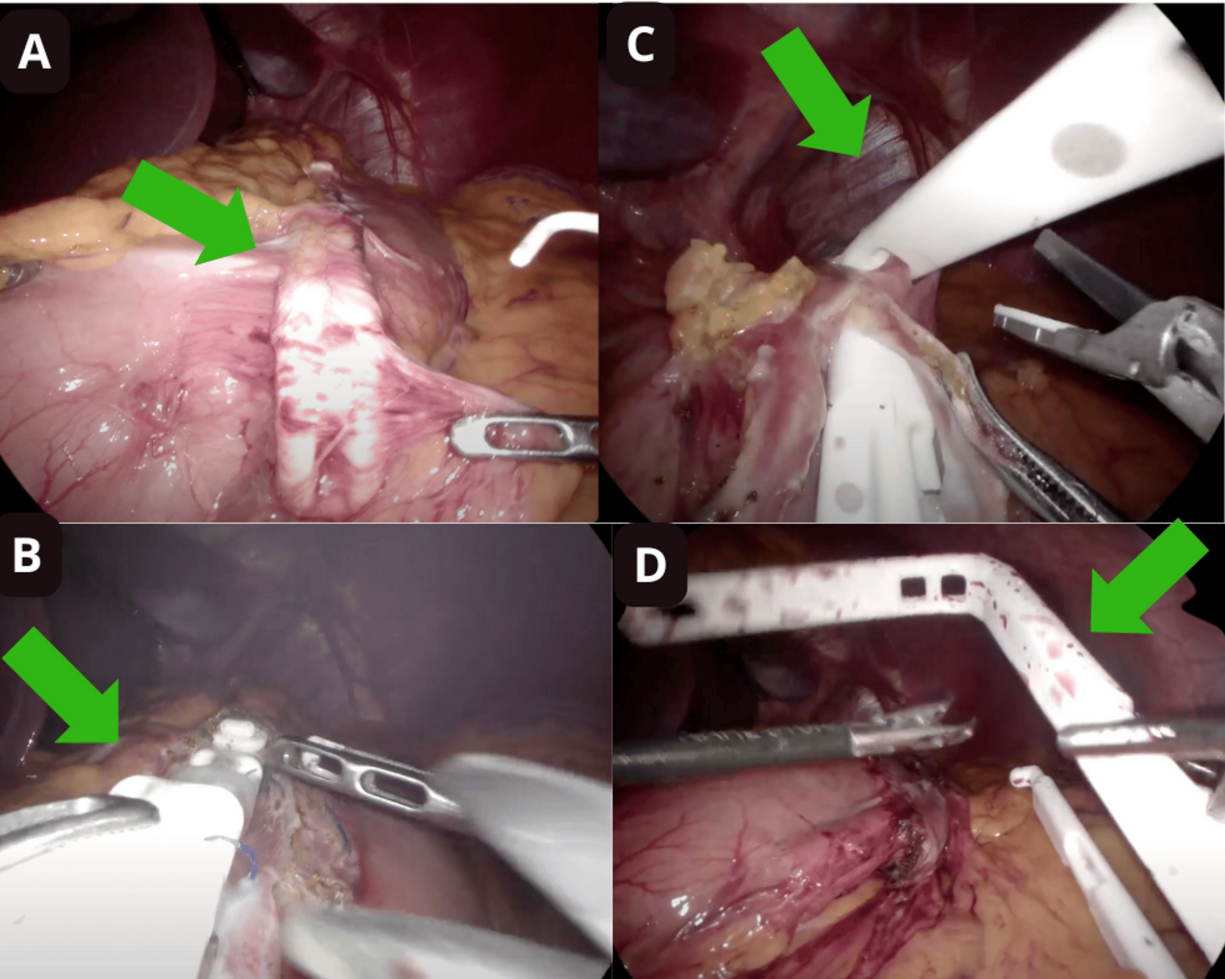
Laparoscopic view in the abdomen. (A) Bariclip in the major curvature of the stomach (green arrow). (B) Liberation of previous sutures of bariclip with ultrasonic energy (green arrow). (C) Liberation of bariclip with ultrasonic energy (green arrow). (D) Bariclip removed (green arrow).
